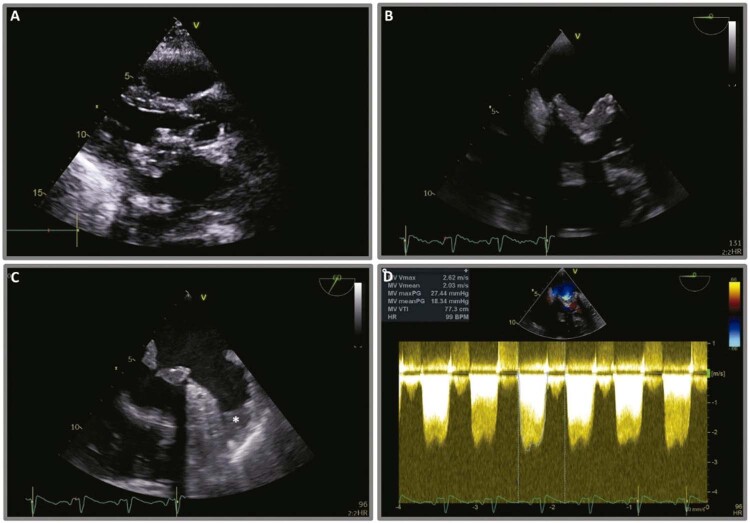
(A) Vista paraesternal eixo longo de ecocardiografia transtorácica basal. (B) Vista ampliada de ecocardiografia transesofágica da valva mitral em 0º e (C) em 60º, confirmando um espessamento anormal dos folhetos (*denota um trombo no apêndice atrial esquerdo). (D) Doppler de onda contínua revelando gradiente médio de pressão transvalvar de 18 mmHg.
